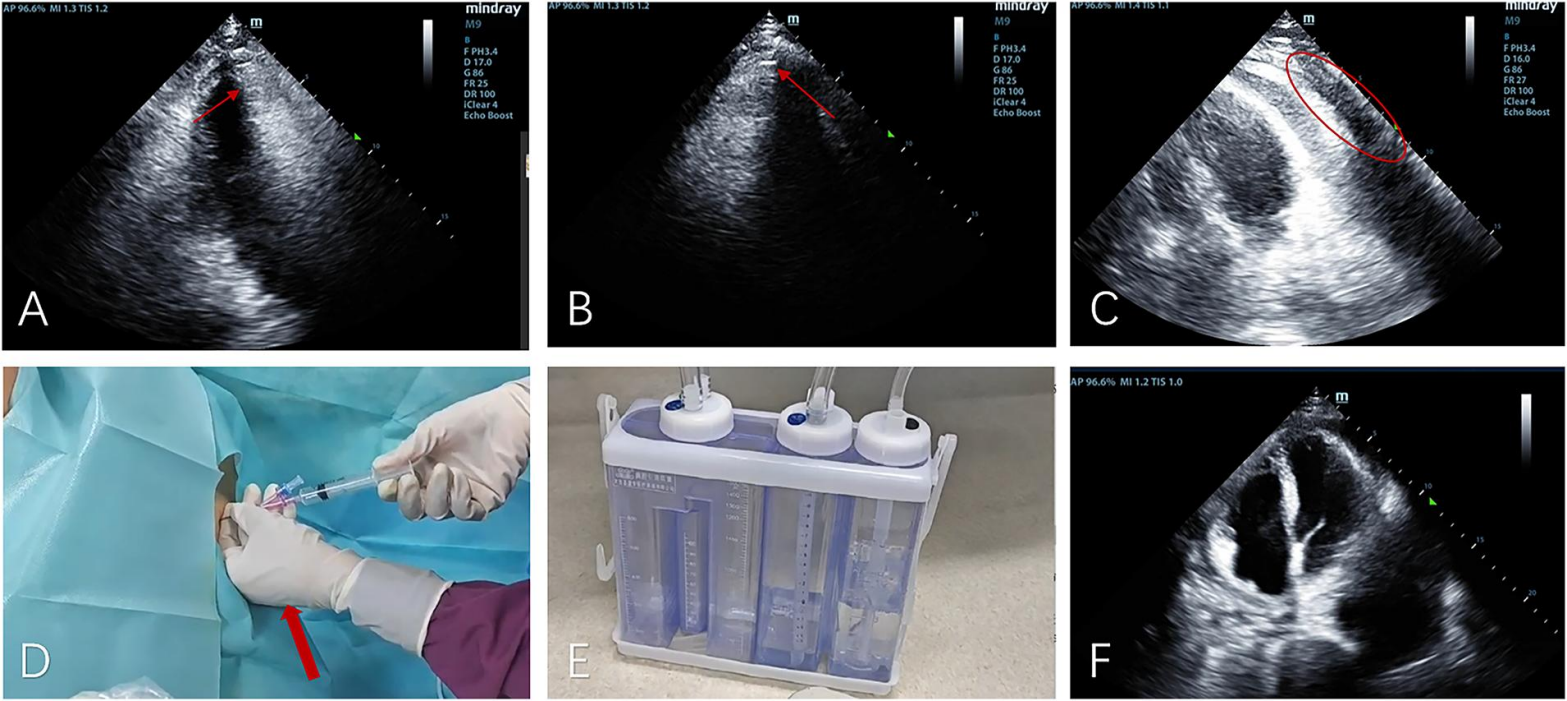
The apical five-chamber view (A), non-standard apical view (B), and apical four-chamber view (C) demonstrate superior displacement of the heart with increased intrapericardial gas compared to Figure 2, showing characteristic air-gap artifacts and air-fluid levels (indicated by yellow arrows). Notably, the swirling microbubble density has increased while gas volume decreased (marked by red circles). (D) Gas was aspirated from the pericardium (indicated by the red arrow); (E) gas was drained into the negative-pressure drainage bottle; (F) a reexamination 4 h after drainage showed a significant reduction in intrapericardial gas.
